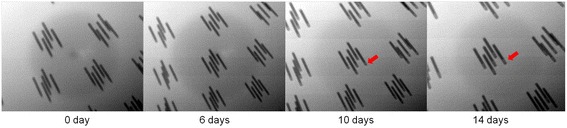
X-ray images showing queue time after Cu seed layer deposition at 10 μm × 100 μm TSVs [45]
